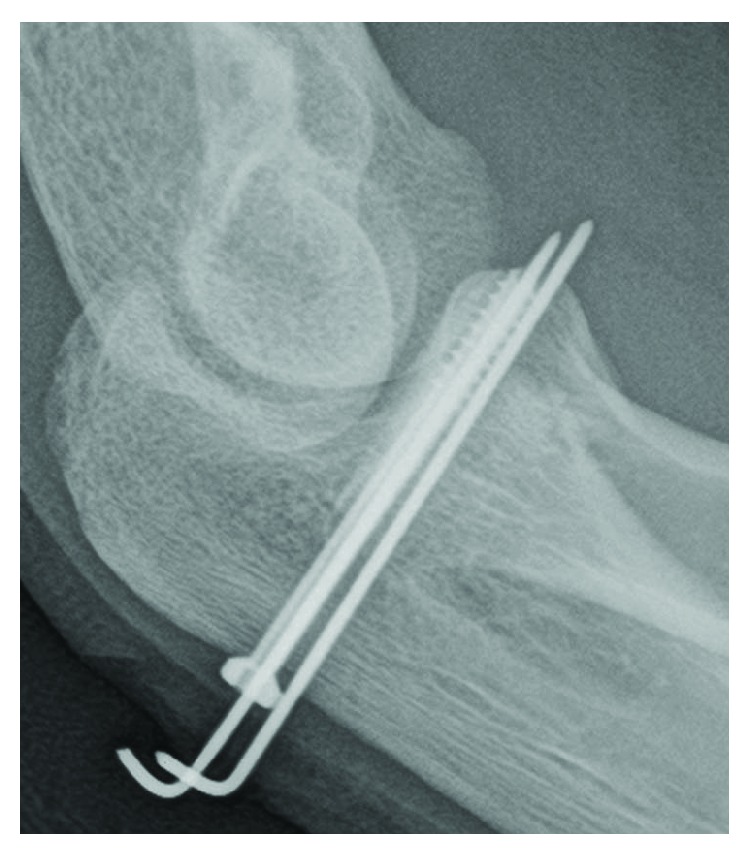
Case 1: postoperative radiograph shows a good reduction position.
